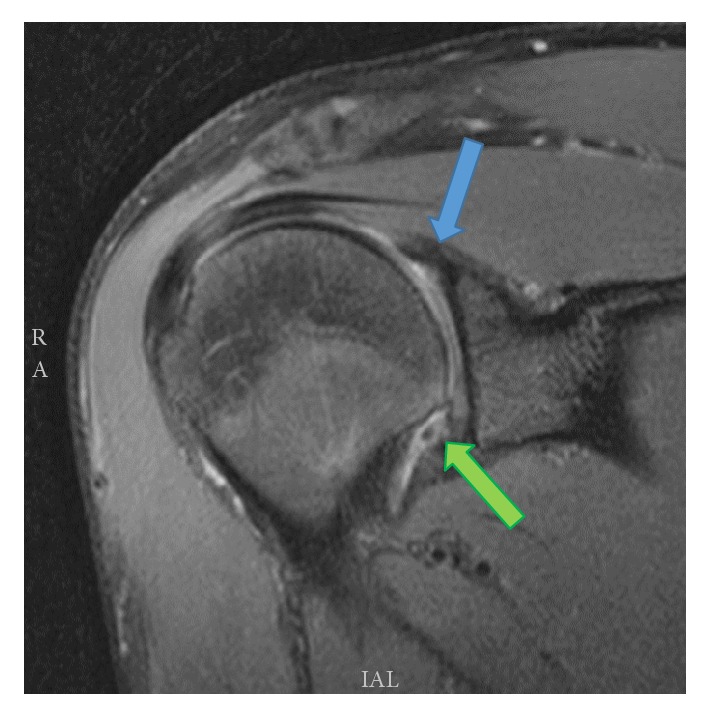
Coronal T2 weighted MRI demonstrating absence of the long head of the biceps tendon (blue arrow) and inferior labral tear (green arrow).
